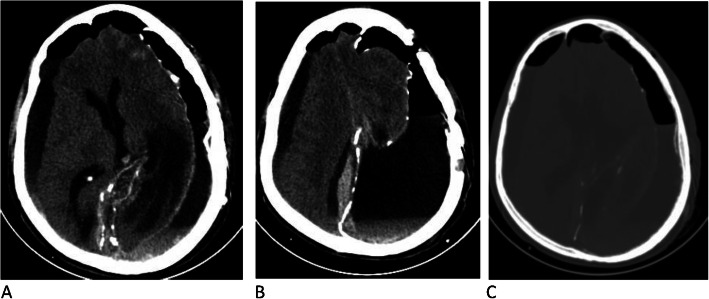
Noncontrast computed tomographic images obtained 48 hours after surgery. Brain window shows bilateral frontoparietal convexity air–fluid level with left parietal hypodensity and mass effect on the left posterior horn of the lateral ventricle (a and b). Bone window shows the bilateral pneumocephalus (c)
